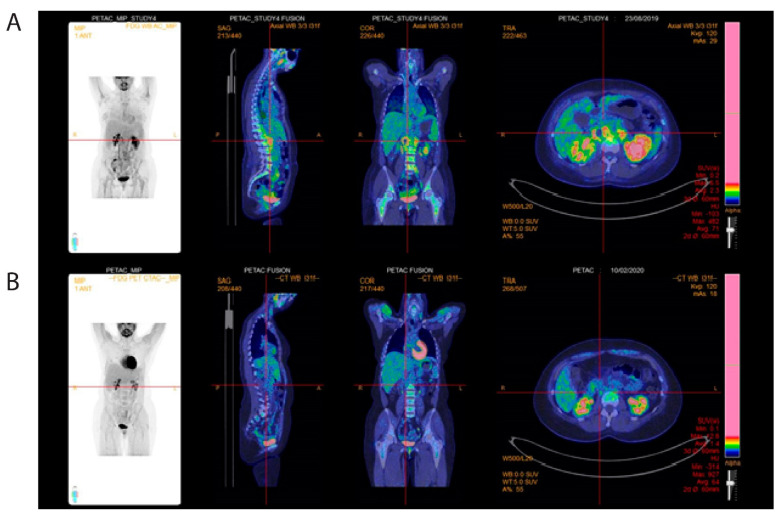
Posttreatment 18-fluorodeoxyglucose (FDG) positron emission tomography (PET)-computed tomography (CT) of abdomen. (A) [^18^F]-FDG-PET/CT pre-therapeutic FDG-positive para-aortic masses. (B) After treatment showing metabolic complete remission.
